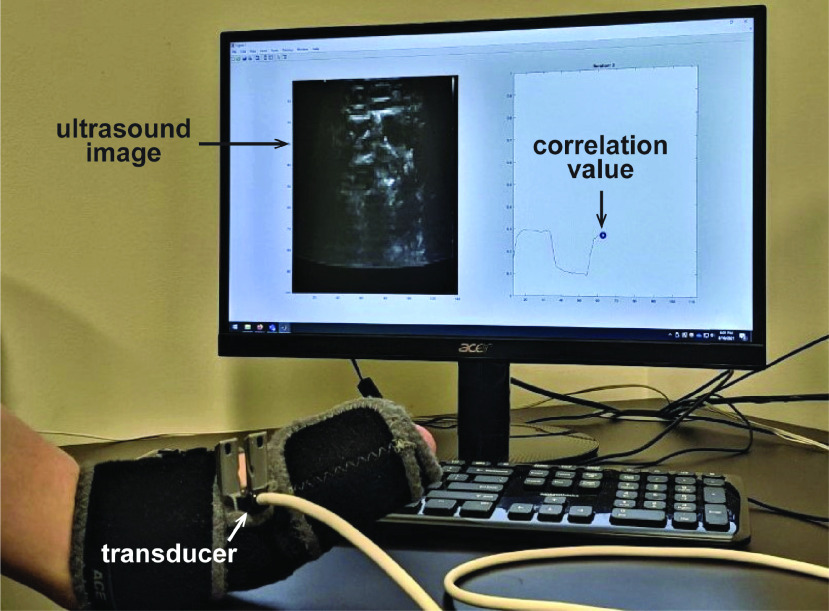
Visual display used to provide feedback to participants during Experiment 2. The Pearson correlation between the current ultrasound image and the first image recorded in the sequence is indicated by the blue circle. High correlation values indicate that current image is dissimilar from rest, while low values indicate similarity to rest. Correlation values for previous images remained on the display (red line) to help participants achieve consistency in the muscle deformation pattern.
